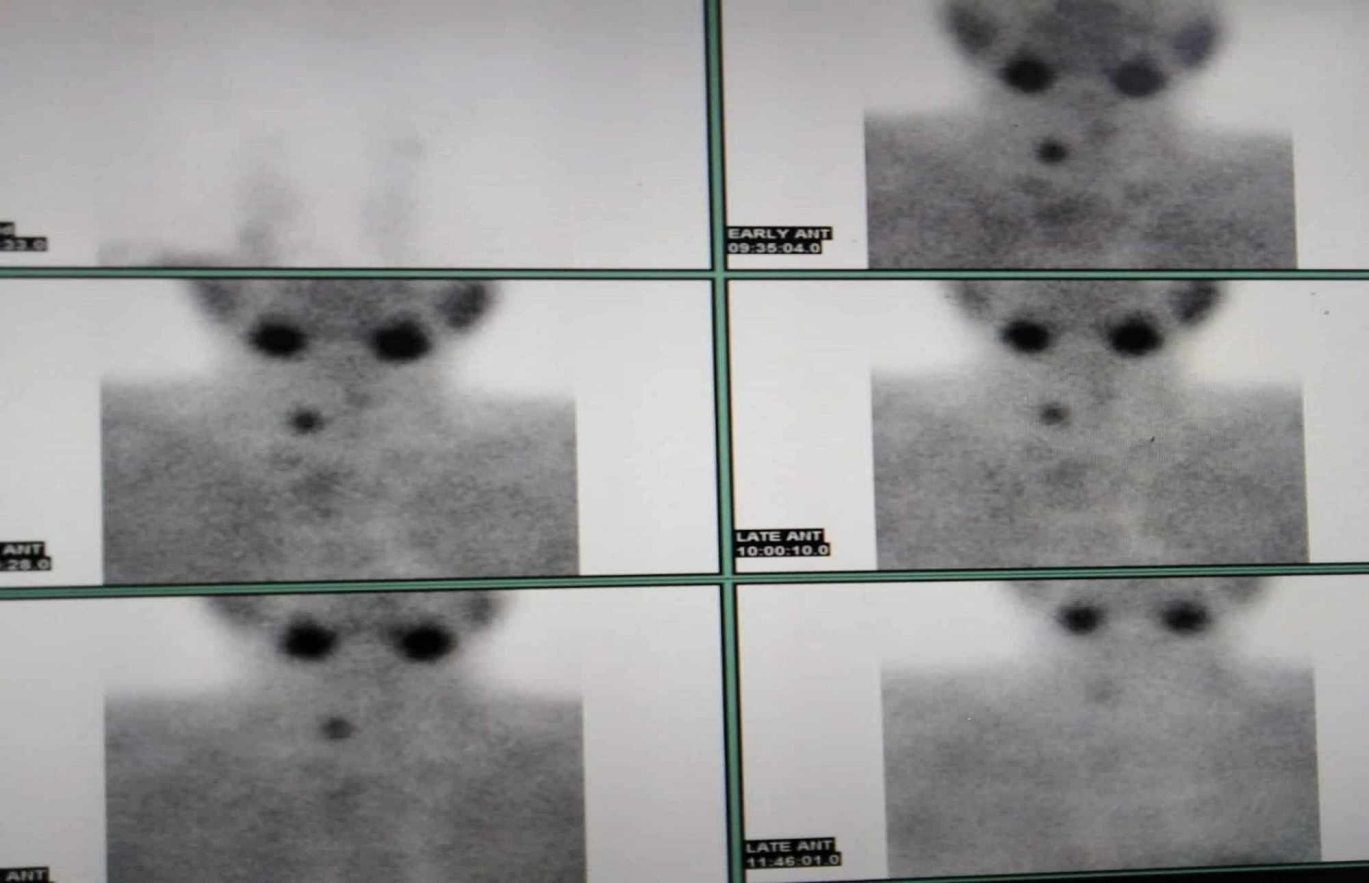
A female patient who complained of bony pains and had a parathormone level of 1045 underwent 99mTc-MIBI parathyroid scintigraphy that showed a rounded area of increased parathyroid activity at the lower right neck (early images) and persisted, becoming more obvious in time, denoting parathyroid adenoma.
